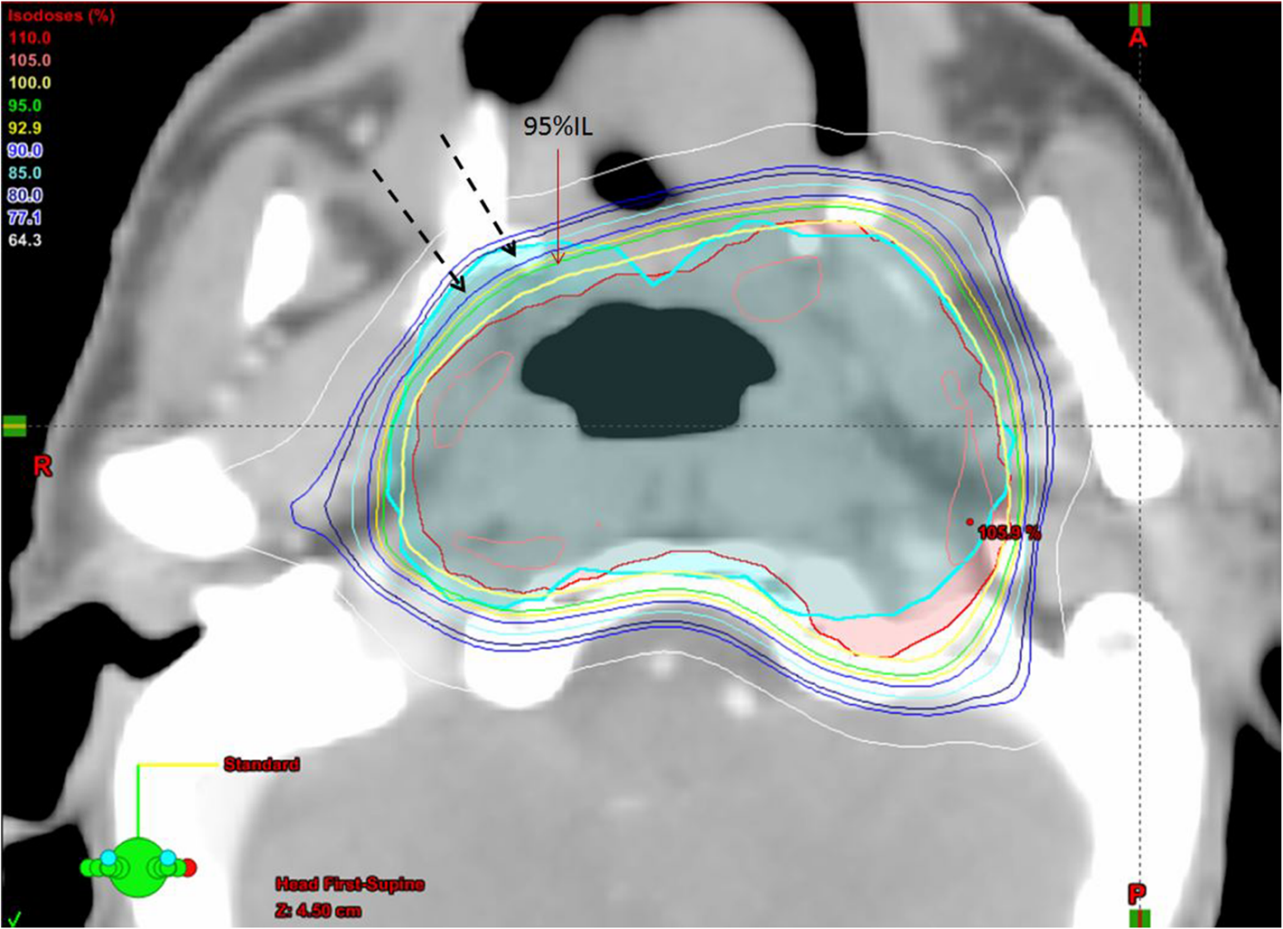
An example of IMRT plan showing the dose distribution at one of the CT slices. The plan was computed based on the PTVCM (Red). The black arrows indicate the regions of PTV_CMP_ (Cyan) receiving less than 95 % isodose level (indicated by the red arrow)
